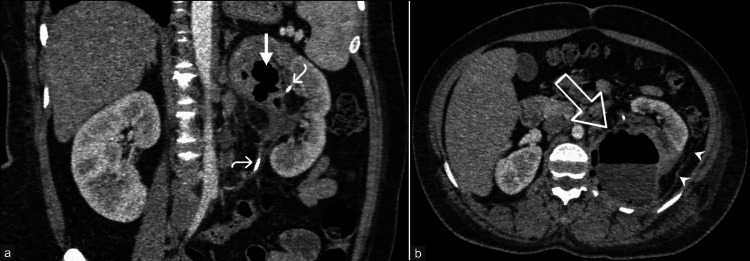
Complicated emphysematous pyelonephritis with renal abscess formation. A 38-year-old woman with a history of ureteroscopic lithotripsy and complaints of left loin pain was diagnosed with complicated acute emphysematous pyelonephritis. (a) Coronal reformatted image of nephrographic phase CT urogram shows relatively enlarged left kidney with architectural distortion, the heterogeneous enhancement of left renal parenchyma with reduced contrast uptake in the affected upper pole, and multiple air pockets (straight arrow) in the parenchyma and pelvicalyceal system. Portions of the proximal end of the double-J stent (curved arrows) can be seen in the left kidney. (b) Axial nephrographic phase CT image shows the presence of a well-defined collection (see-through arrow) epicentered in the upper pole of the left kidney, demonstrating air-fluid level and rim enhancement. The thickening and enhancement of the posterior renal fascia (arrowheads) is also seen. These images are from the hospital and have not been published elsewhere. CT: computed tomography
